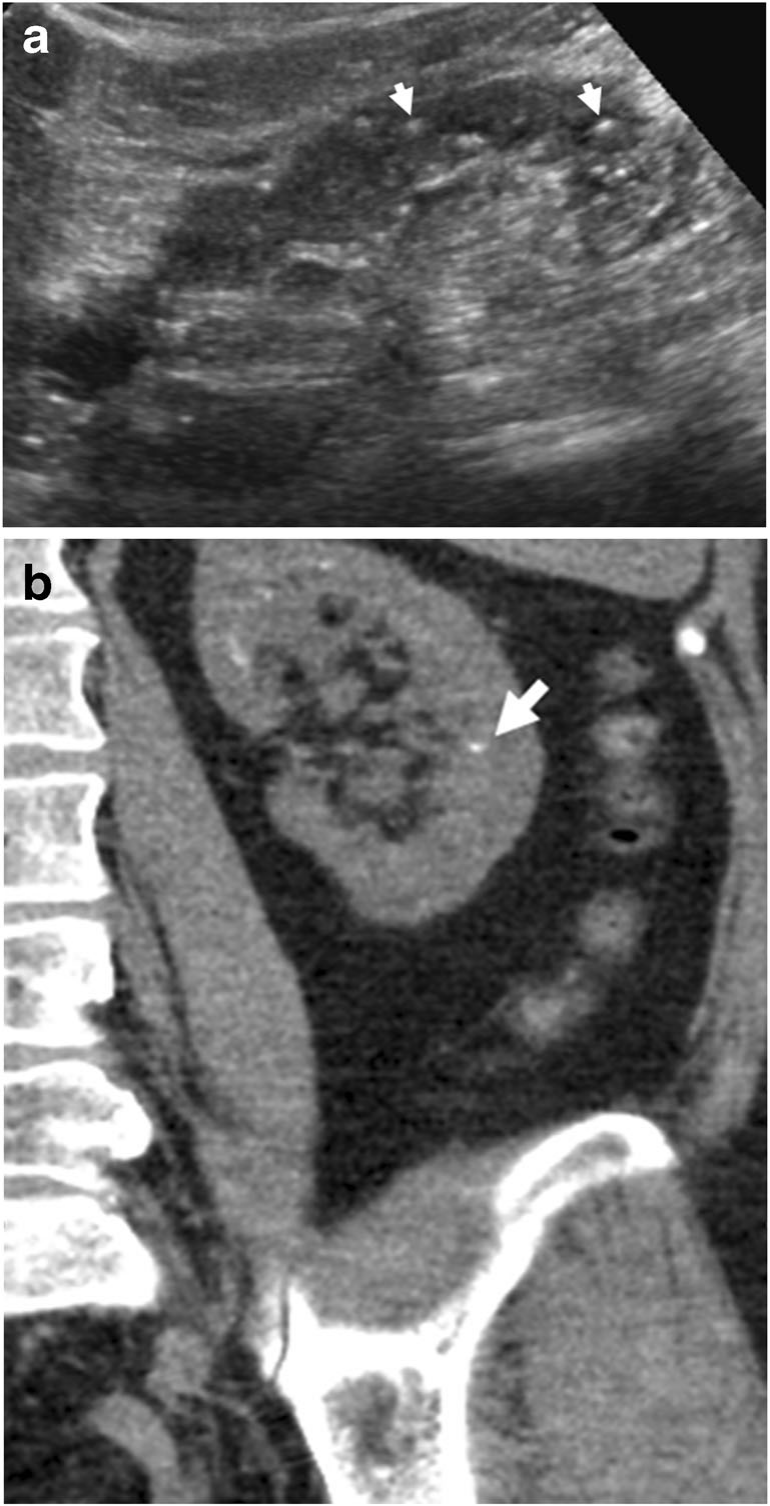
a–b: a Grey-scale US and (b) unenhanced CT, coronal reformations. Papillary calcifications in a patient undergoing long-term dialysis, appearing as hyperchoic spots on renal pyramids. Papillary calcifications may also be observed in analgesic nephropathy, in sarcoidosis, primary hyperparathyroidism, diabetes mellitus and medullary sponge kidney
